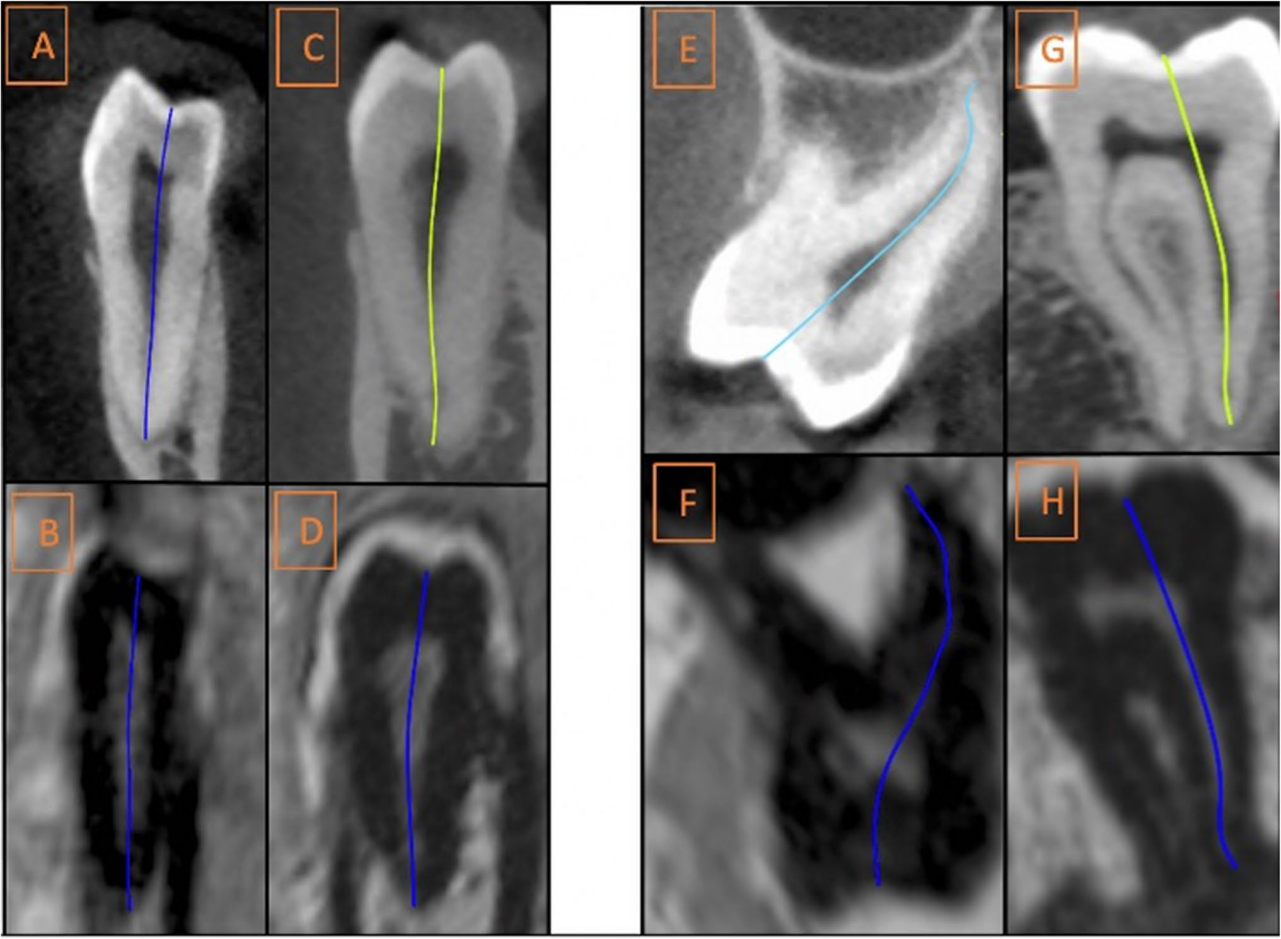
Working length measurements in CBCT and dMRI for premolars (left, A–D) and molars (right, E–H); a first left lower (A, B) and first right lower premolar (C, D); a second left upper molar (E, F) and second left lower molar (G, H)
